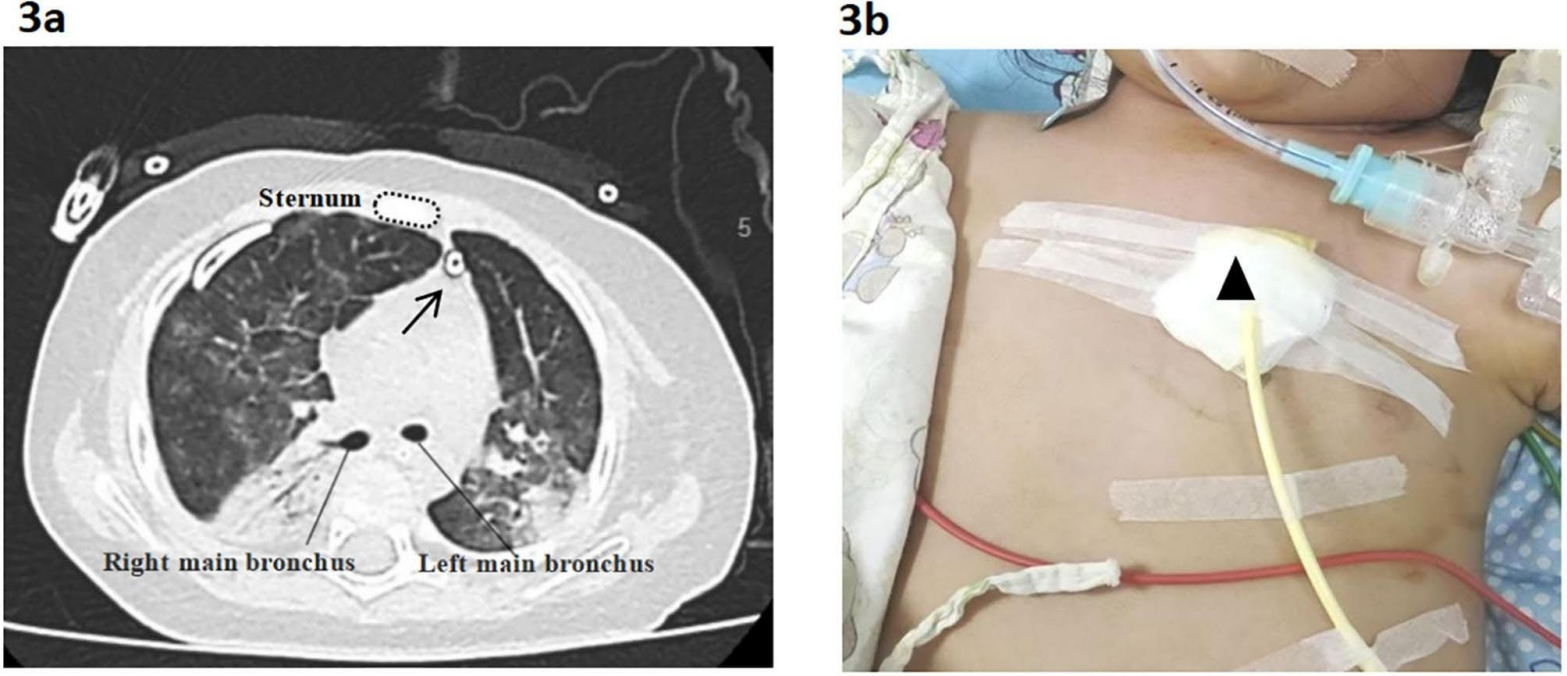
3a: Postoperative chest CT showed a satisfactory location of the pigtail catheter in the anterior mediastinum. (→). 3b: The place to insert the pigtail catheter.(▲)
